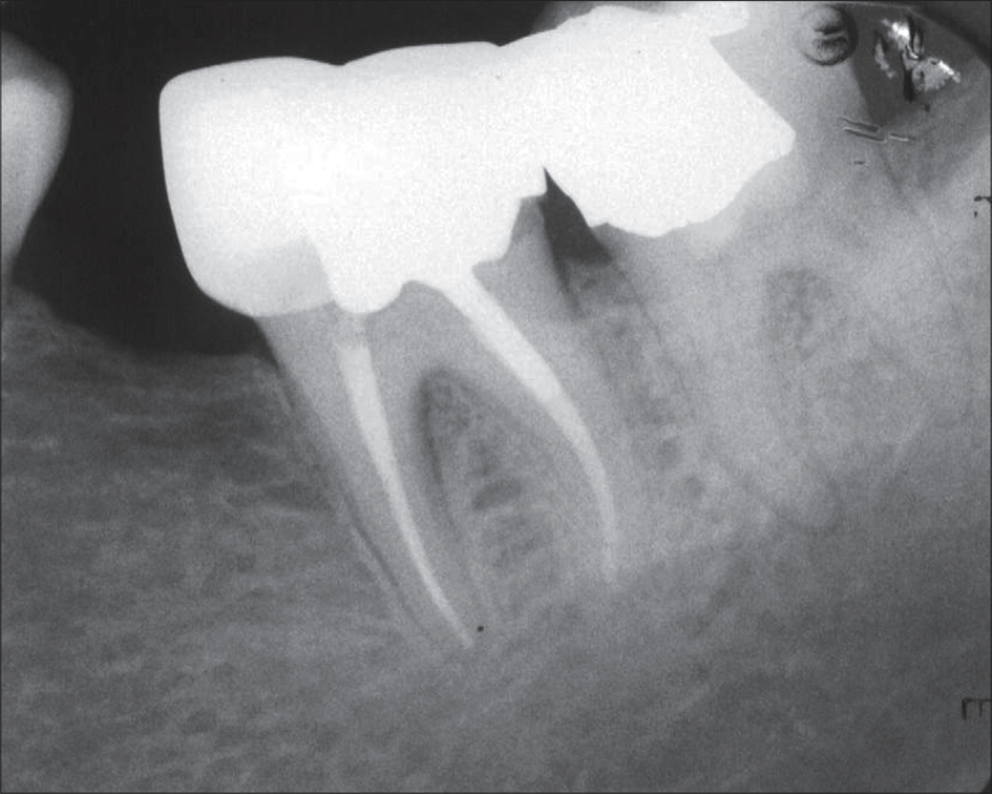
Twelve-month follow-up radiograph showing favorable outcomes. The treated teeth are in function and the periapical tissues are normal. An important finding is the complete healing of the periradicular lesion in the mesial root of the third molar
